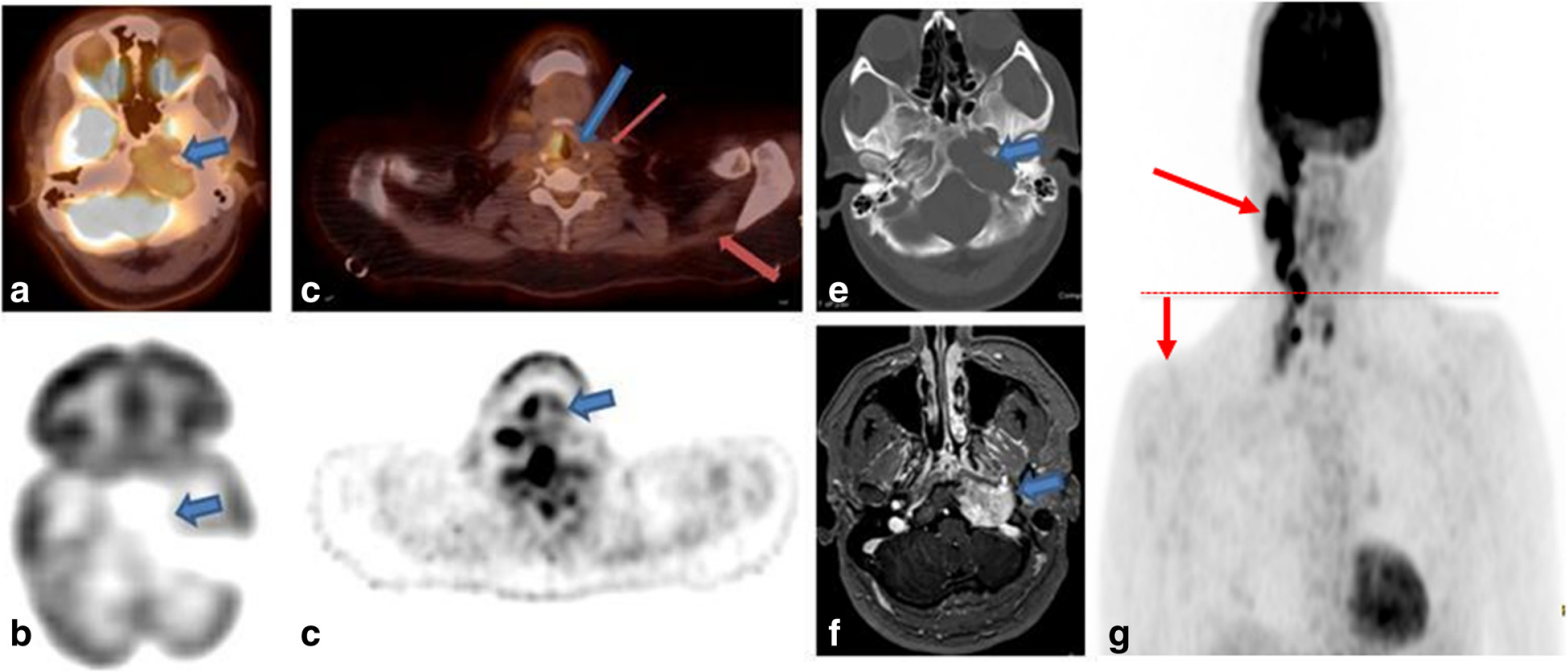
A 32 year-old-female presenting with a hoarse voice. Axial fused (a) and unfused (b) PET/CT images of the skull base showing a hypometabolic soft tissue mass centered on the left jugular foramen with smooth osseous expansion suggestive of schwannoma of cranial nerve IX, X or XI which all exit the skull base through the jugular foramen. Axial fused (c) and unfused (d) PET/CT images at the level of the glottis show no 18F-FDG uptake in the left vocal cord (blue arrow) with compensatory increased activity in the right vocal cord, consistent with laryngoscopy proven left vocal cord paralysis due to tumor involvement of left CN X and its recurrent laryngeal branch. Asymmetric atrophy of the left sternomastoid and trapezius muscles is consistent with chronic denervation due to tumor involvement of the left cranial nerve XI (spinal accessory nerve) (red arrows). Axial CT scan of the skull base with bone window settings (e) and axial post-contrast fat-suppressed T1 MRI (f) at the same level show the enhancing left cranial nerve IX/X/XI mass pathologically proven to be a schwannoma. g Coronal MIP image of a 50-year-old male with HIV presenting with worsening right facial weakness and pathologically proven squamous cell carcinoma of the neck, with perineural tumor invasion along the jugular foramen (not shown), showing right shoulder drooping compared to the left one (red line), secondary to atrophy of the right trapezius and sternomastoid muscles, confirmingCN XI involvement. Also note the bulky hypermetabolic cervical adenopathy (arrow) in the rightneck involving lymph node levels 1 through 4 consistent with metastatic lymph nodes
